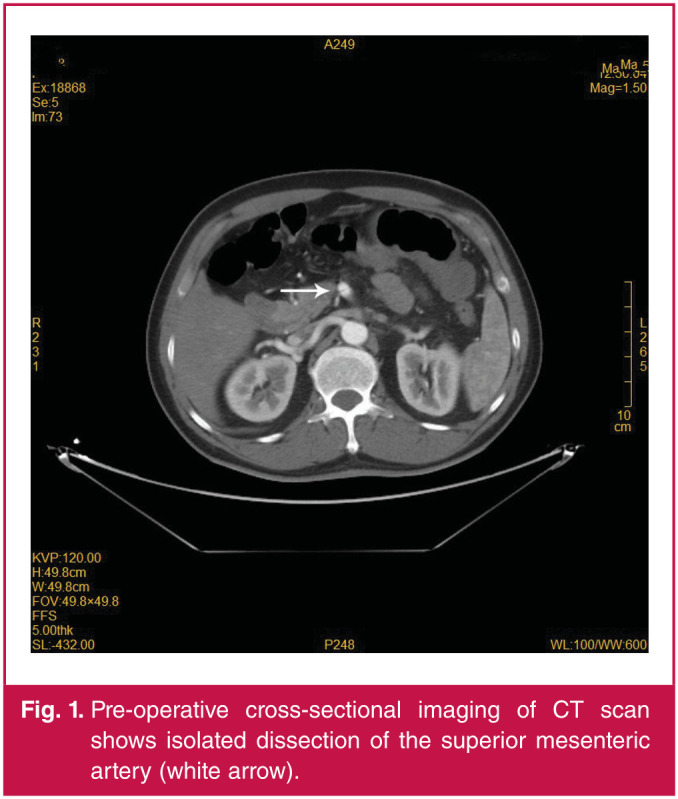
Pre-operative cross-sectional imaging of CT scan shows isolated dissection of the superior mesenteric artery (white arrow).
